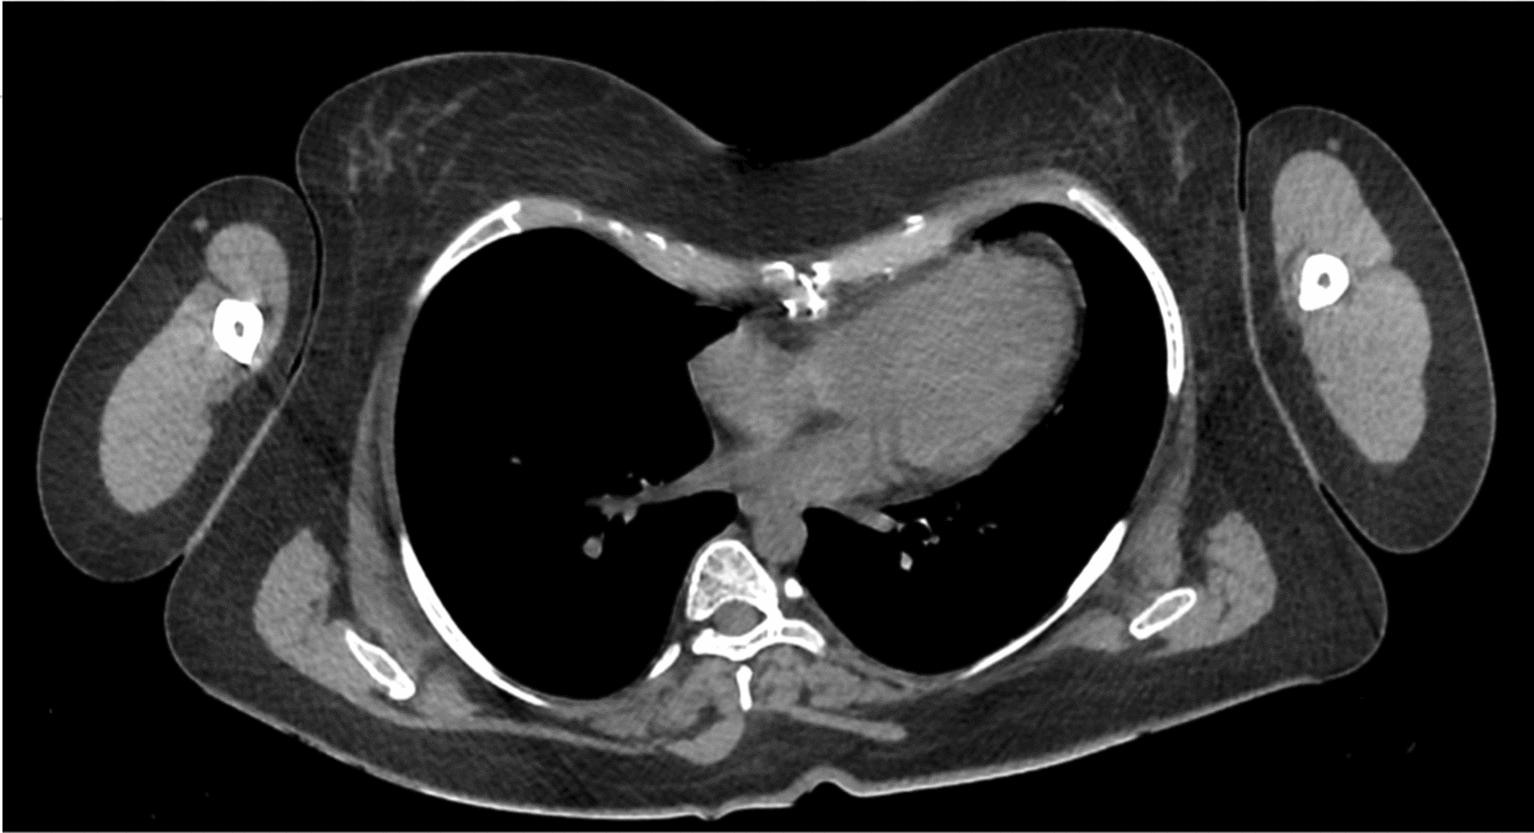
Axial computed tomography slice (inferior view) showing a rotated vertebral column protrusion into the right thoracic cavity in an Osteogenesis Imperfecta patient
